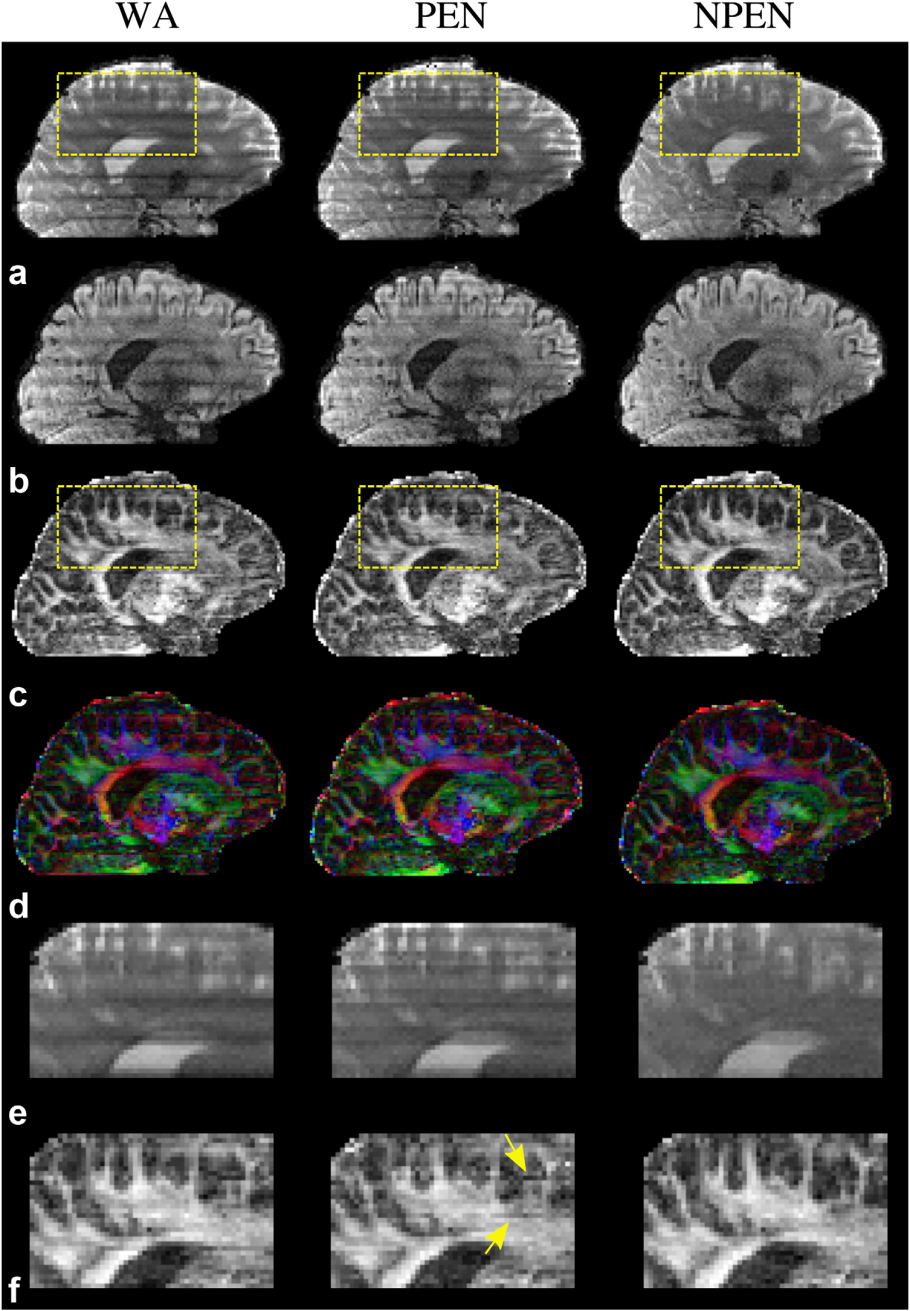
Comparison of three reconstruction methods based on in vivo data. (a) b = 0 image. (b) DWI image (b = 1000 s/mm^2^). (c) FA maps. (d) Color‐coded maps of the principal eigenvector (red, right–left; green, anterior–posterior; blue, superior–inferior). (e, f) Zoomed‐in regions specified by the yellow rectangles in panels a and c, respectively.
